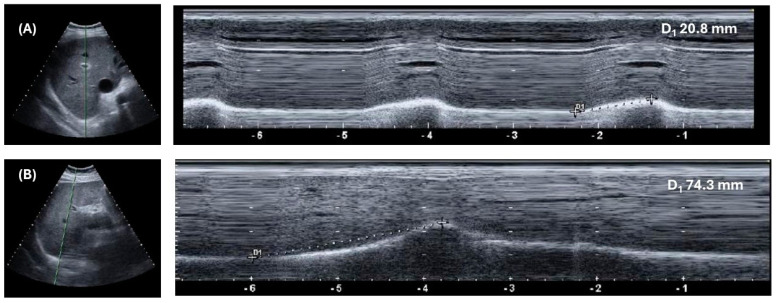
Ultrasonographic measurement of diaphragm excursion in an older subject with heart failure on quiet breathing (A) and maximal voluntary inspiration (B). The diaphragm is visualized in the B-mode subcostal scan as the hyperechoic cupola-like line surrounding the liver parenchyma. The movements of this line on quiet breathing (A) and maximal voluntary inspiration (B) are then assessed activating the M-mode ultrasound functionality, which allows us to visualize peaks in the hyperechoic line correspondent to the diaphragm, synchronous with breaths. The height of these peaks corresponds to diaphragm excursion.
